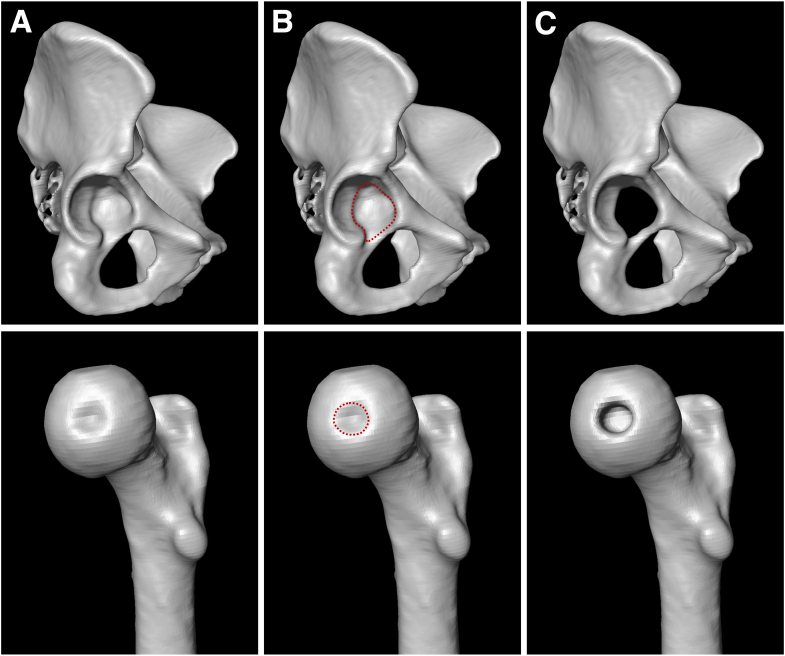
(A) We built a 3-dimensional model from preoperative computed tomography scans of each patient. (B) The fossa and fovea are identified and marked. (C) The fossa is excised out of the acetabulum, and the fovea is manually recessed by approximately 10 mm.
